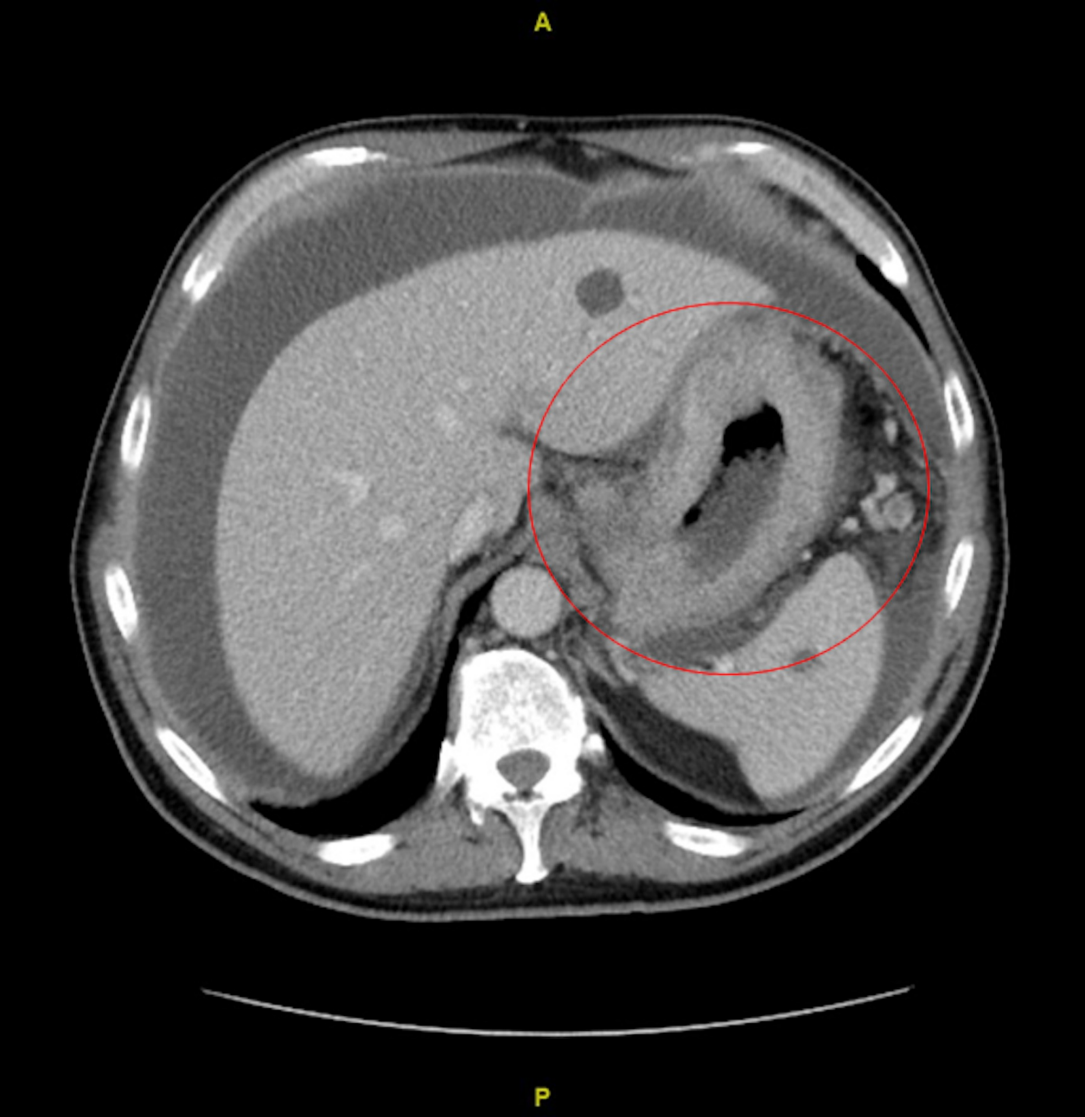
CT Abdomen and Pelvis (axial view) demonstrating gastric wall thickening and significant ascites. Incidental cysts can be visualized in the liver and spleen.
